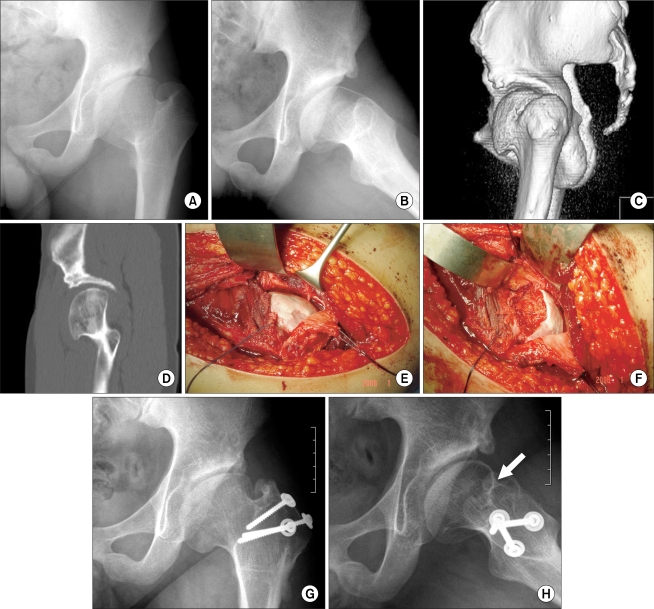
A 16-year-old boy with Legg-Calvé-Perthes disease of the left hip was treated with femoral valgization osteotomy 6 years ago and shelf acetabuloplasty 3 years ago. He had limited left hip flexion and a positive impingement test (A). Lauenstein view (B), 3D-CT (C), and sagittal reconstruction view (D) revealed an anterior hump of the femoral head causing cam-type femoroacetabular impingement, which was also confirmed in the subluxated femoral head using the Ganz surgical hip dislocation approach (E). Osteochondroplasty relieved the impingement (F). Plain radiographs taken 1.5 years later showed a well-formed head-neck offset (white arrow) (G, H). Hip flexion range improved from 95° in the preoperative period to 130° at 1.5 years follow-up.
